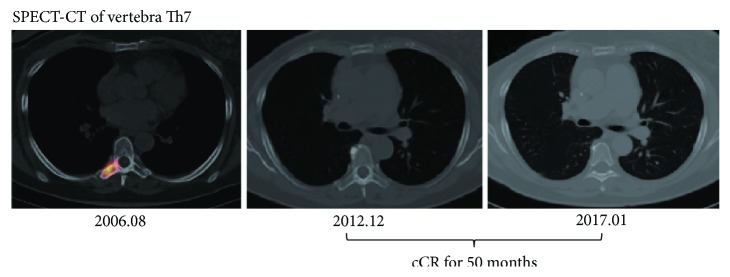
Follow-up SPECT-CT of vertebra Th7 of Case #6 (assigned as cCR Case 1) [20]. No recurrence in vertebra Th7 was observed.
